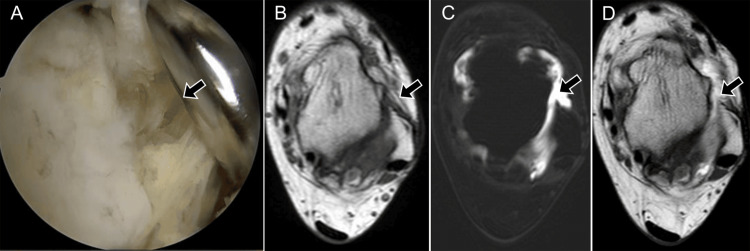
Chronic anterolateral ankle instability - arthroscopy and MRI. A 45-year-old female: (A) arthroscopic view via an ATFL approach shows absence of ATFL fibers (arrow); (B) axial T2 MRI without fat suppression shows irregular, thickened ATFL with mild hyperintensity (arrow); (C) post-contrast axial T1 fat-saturated MRI shows contrast leakage into the anterolateral recess (arrow); (D) axial T1 spin-echo MRI confirms ligament discontinuity (arrow). ATFL: anterior talofibular ligament Images belong to the authors; patient consent was obtained.
